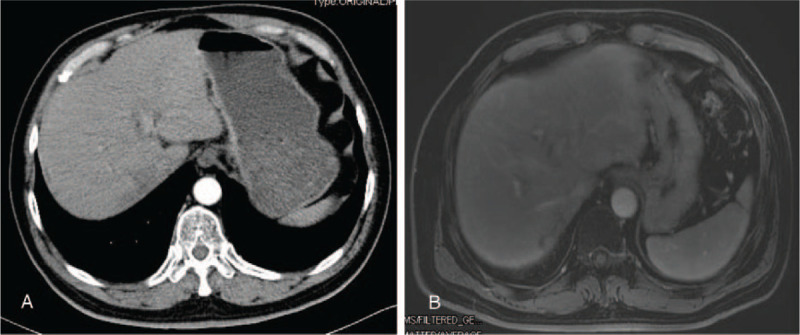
Dynamic contrast-enhanced CT (A) and MRI (B) scan findings after SBRT combined with sorafenib therapy (2 months later). The early phase of dynamic contrast-enhanced CT (A) and MRI (B) showed that the vascularity of the intrahepatic mass had been lost. No thrombus was found in the right hepatic vein or IVC. IVC = inferior vena cava, MRI = magnetic resonance imaging.
